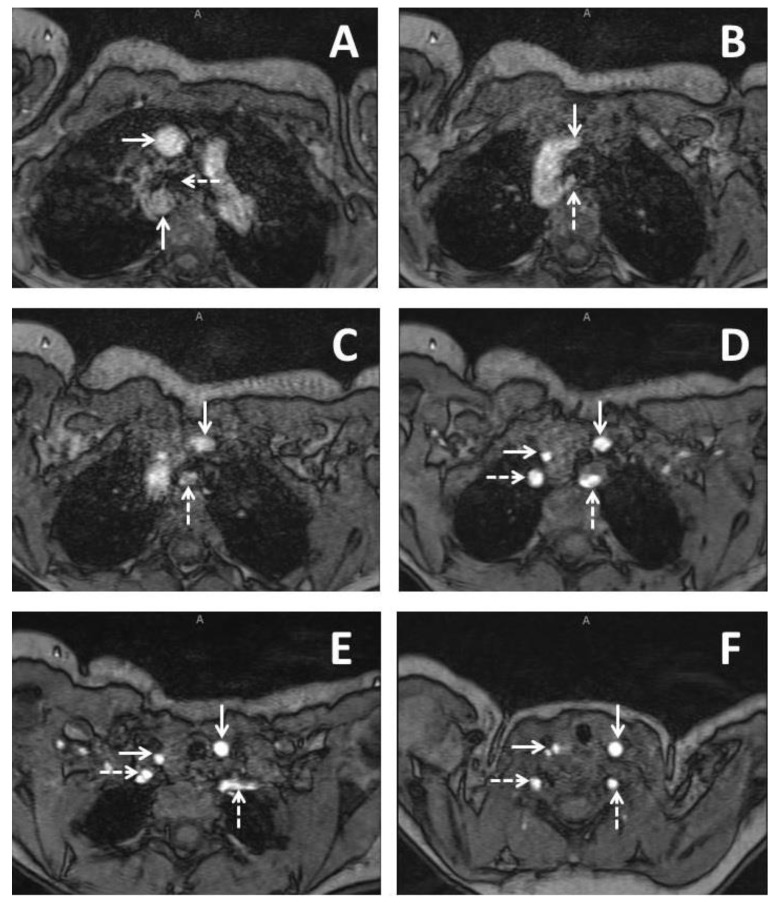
Imaging from a three months old baby girl with DiGeorge syndrome who had some issues with feeding. Series of axial slices from magnetic resonance imaging presented in an inferior to superior progression. Panel A demonstrates a right sided aortic arch with the ascending aorta (solid horizontal arrow) coursing anterior to the right bronchus (dashed horizontal arrow) and the descending aorta (solid vertical arrow) coursing immediately posterior to the right bronchus; Panel B demonstrates the left common carotid (solid vertical arrow) as the first branching head and neck vessel and arises from the ascending aorta. An aberrant left subclavian artery (dashed vertical arrow) is seen as the last branching head and neck vessel and arises from the descending aorta; Panel C demonstrates the course of the left common carotid (solid vertical arrow) and aberrant left subclavian artery (dashed vertical arrow); Panel D demonstrates bifurcation of the brachiocephalic trunk into the right common carotid (solid horizontal arrow) and the right subclavian artery (dashed horizontal arrow). The left common carotid (solid vertical arrow) and the left subclavian artery (dashed vertical arrow) are seen demonstrated as well; Panel E demonstrates the right vertebral artery arising from the right subclavian artery (dashed horizontal arrow). The right common carotid (solid horizontal line), left common carotid (solid vertical arrow, and aberrant left subclavian (dashed vertical arrow) are also demonstrated; Panel F demonstrates early bifurcation of the right common carotid into the right internal and external carotid arteries (solid horizontal arrow). The right vertebral artery (dashed horizontal arrow), the left common carotid (solid vertical arrow), and the left vertebral artery are also demonstrated (dashed vertical arrow).
